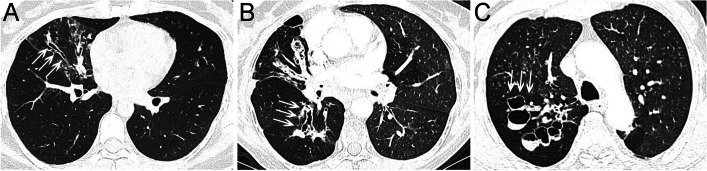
Computed tomography images of the three types of bronchiectasis. A Columnar bronchiectasis (white arrows); B Varicose bronchiectasis (white arrows); C Cystic bronchiectasis (white arrows)
